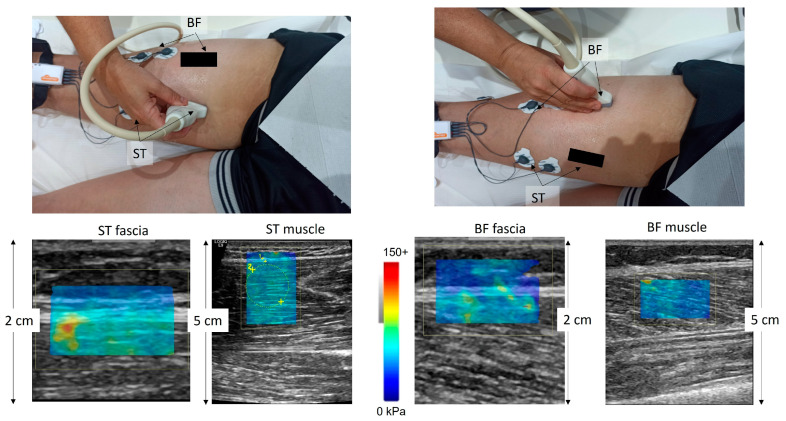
The experimental set-up. The ultrasound probe was placed on the semitendinosus (ST) and the biceps femoris long head (BF) at 40% of the distance from the ischial tuberosity to the distal ends. Elastograms were superimposed on B-mode images as color-coded boxes. For fascia tissue analysis, the images were zoomed so that the fascia tissue was enlarged and separated from the irrelevant tissues. Circles were then drawn inside the fascia or the muscle tissue, and the shear modulus values were calculated. The color scale was extracted from the software and is enlarged so that the measurement scale is easily visible.
